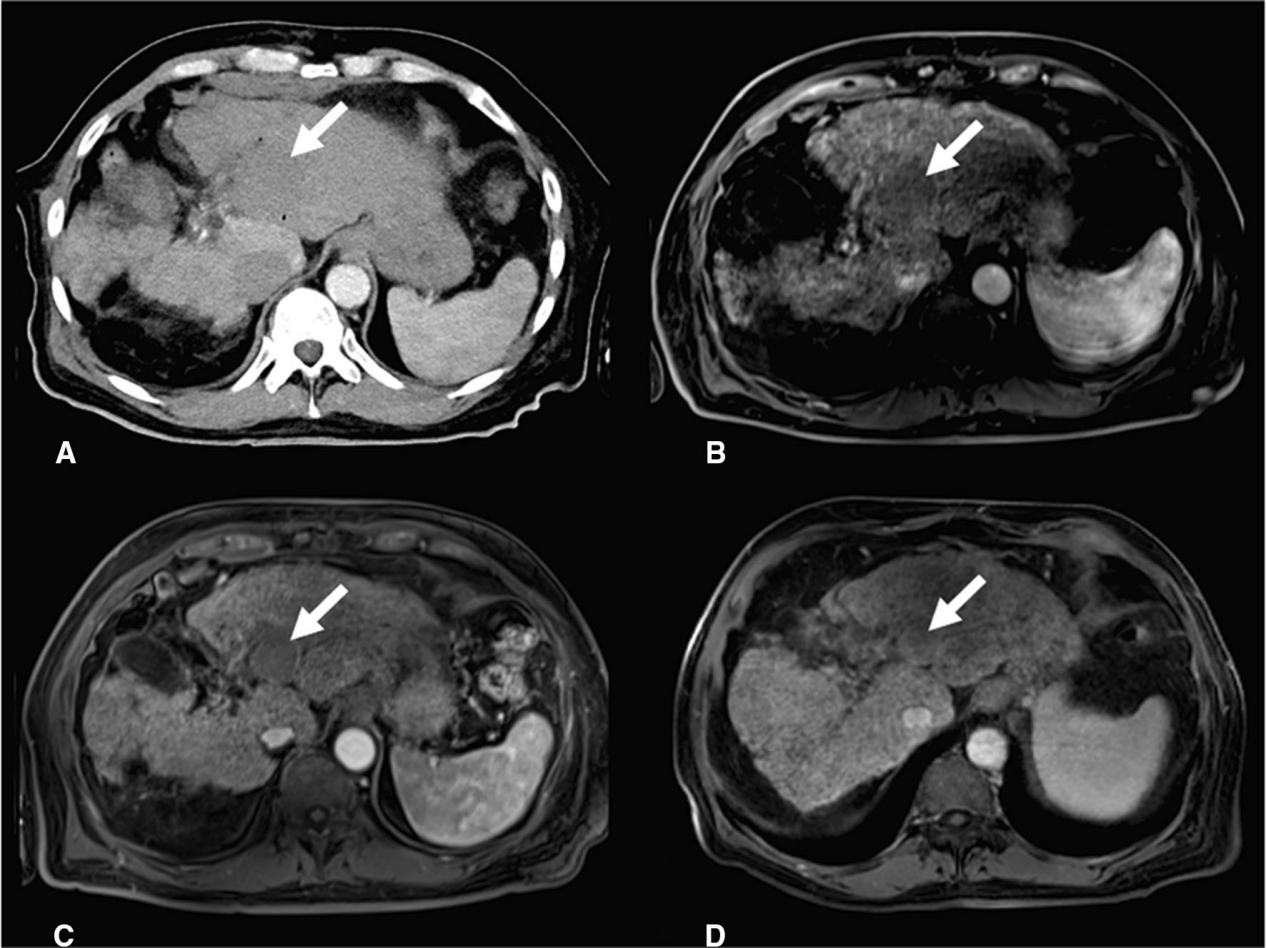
Follow-up imaging. A Contrast-enhanced CT scan in arterial phase at the end of intervention shows complete devascularization of the tumor (arrow). B Contrast-enhanced MRI in arterial phase, T1 Vibe 3d fs, 24 h after ablation confirms complete tumor devascularization (arrow). C and D Contrast-enhanced MRI in arterial phase, T1 Vibe 3d fs, 6 weeks (C) and 6 months (D) after ablation show complete response according to mRECIST and shrinking of the ablation defect to 3.1 × 2.8 × 2.8 cm without affection of the liver arteries and with almost complete restoration of the local cholestasis (arrow)
